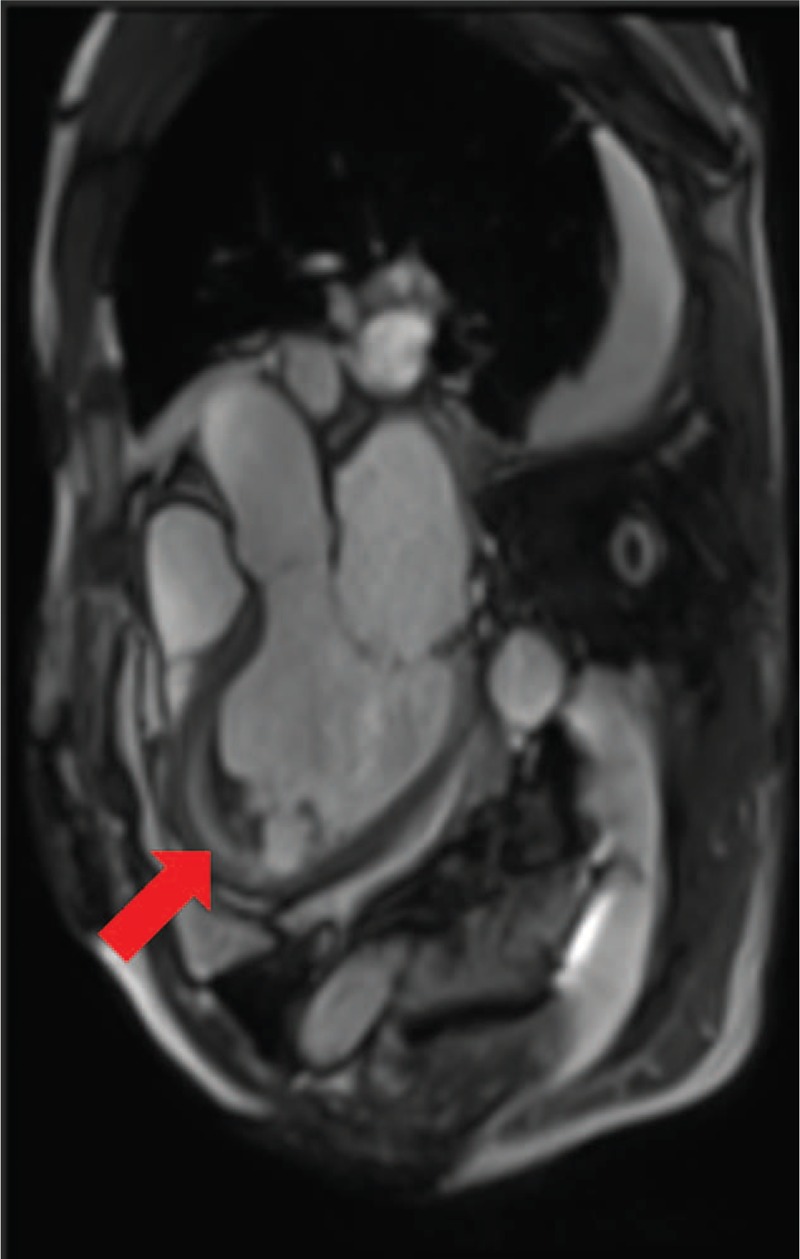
MR image of heart showed intraventricular thrombus (arrow) and thin endocardium with high-intensity signal. MR = magnetic resonance.
